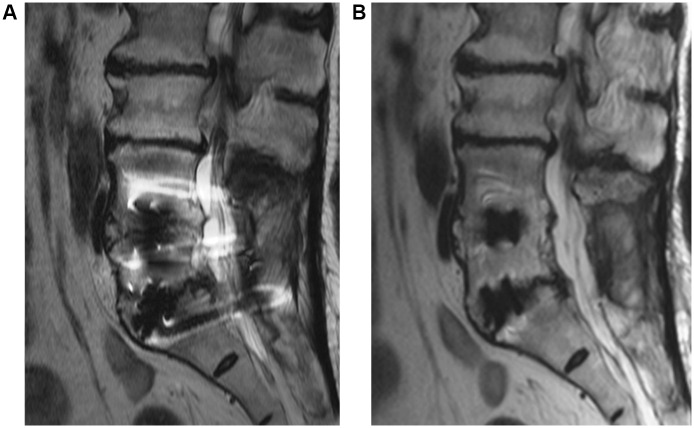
Visibility score of bone-cage interface and diagnostic confidence of inter-body non-union in sagittal images. These two sagittal images are obtained from a 72 year old female who underwent inter-body fusion at L4-5 and L5-S1 and posterior instrumentation at L4-S1 level due to spinal stenosis. The time interval between surgery and the spine MRI was 1 years and 3 months. (a) Sagittal Fast spin echo T2 weighted image shows grade 1, 1 (observer 1) and 1, 1 (observer 2) at L4-5 and grade 1, 1 (observer 1) and 1, 1 (observer 2) at L5-S1 of interface between upper and lower margin of the cage and bone marrow. (b) Sagittal FSE-SEMAC T2 weighted image shows grade 3, 2 (observer 1) and 2, 2 (observer 2) at L4-5 and grade 3, 2 (observer 1) and 2, 2 (observer 2) at L5-S1 of interface between upper and lower margin of the cage and bone marrow. Diagnostic confidence level was grade 3 (inconclusive, observer 1) and 2 (suspiciously absent, observer 2) in FSE T2 weighted sagittal image, but after application of FSE-SEMAC, diagnostic confidence level was enhanced to grade 2 (suspiciously absent, observer 1) and grade 1 (definitely absent, observer 2), respectively.
